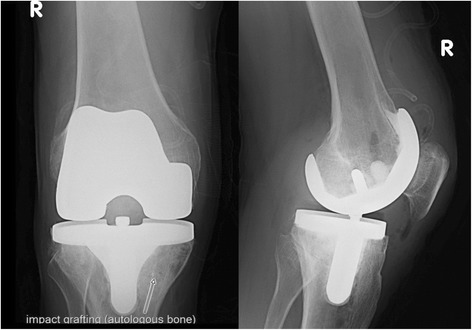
Postoperative X-ray of the same knee with impact grafting of the haemophiliac pseudocyst (cemented standard CR implant)
